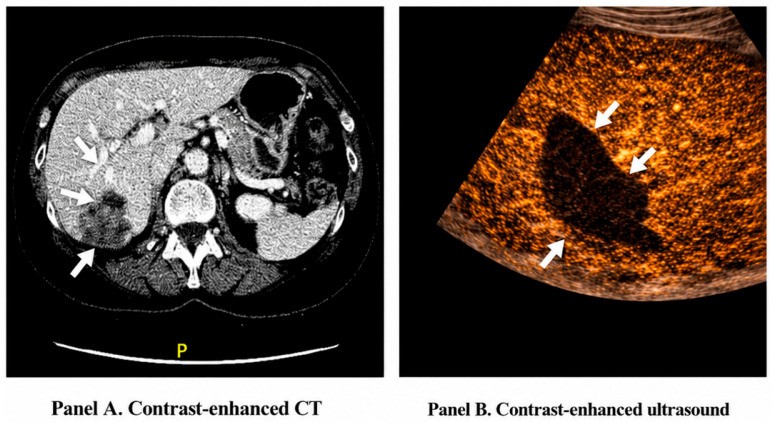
Hepatic infarction: CT and CEUS correlation in a real-world case involving segment VII. Panel (A): contrast-enhanced CT shows a peripheral wedge-shaped hypodense lesion in segment VII (arrows). Panel (B): contrast-enhanced ultrasound demonstrates an avascular, persistently hypoenhancing area corresponding to the infarcted region (arrows). Imaging interpretation is consistent with reported CT and CEUS features of hepatic infarction [10,21,29,30]. The CT and CEUS images are original anonymized clinical images from the Department of Internal Medicine, Morgagni-Pierantoni Hospital, AUSL Romagna, Forlì, Italy.
